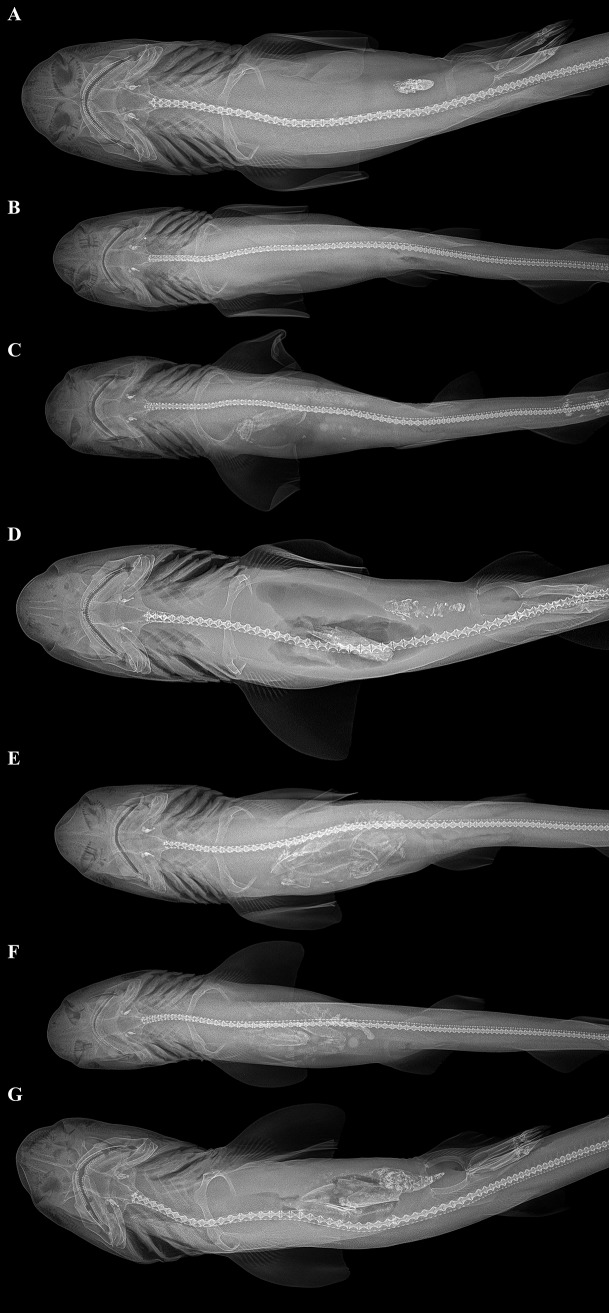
Exemplary radiographs of selected paratypes of *Bythaelurus stewarti* n. sp. in dorsal views depicting different scores of stomach fullness. (A) paratype, ZMH 26254, adult male, 391 mm TL, score 0, (B) paratype, ZMH 26254, juvenile female, 296.4 mm TL, score 0, (C) paratype, ZMH 26252, juvenile female, 299 mm TL, score 1 containing a teleost and few eye lenses, (D) paratype, ZMH 26254, adult male, 417 mm TL, score 1 containing a decapod (probably brachyuran) claw, (E) paratype, ZMH 26252, juvenile male, 340 mm TL, score 2 containing, among other remains, two decapod (probably brachyuran) claws and one teleost, (F) paratype, ZMH 26252, juvenile female, 299 mm TL, score 2 containing two decapod (probably brachyuran) claws and few eye lenses, and (G) paratype, ZMH 26254, adult male, 375 mm TL, score 2 containing a decapod (probably brachyuran) claw and a teleost.
